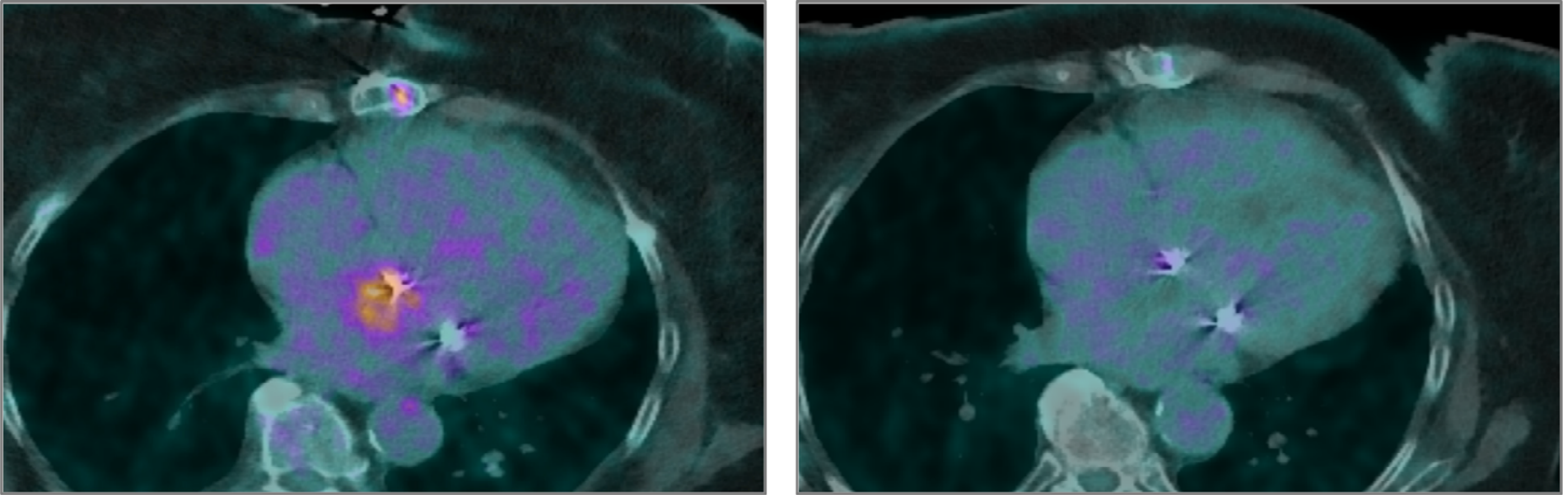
PET scan showing FDG standardised uptake value of 6.1 before treatment (left) and 4.2 two months after treatment (right) in the left atrial lesion.
